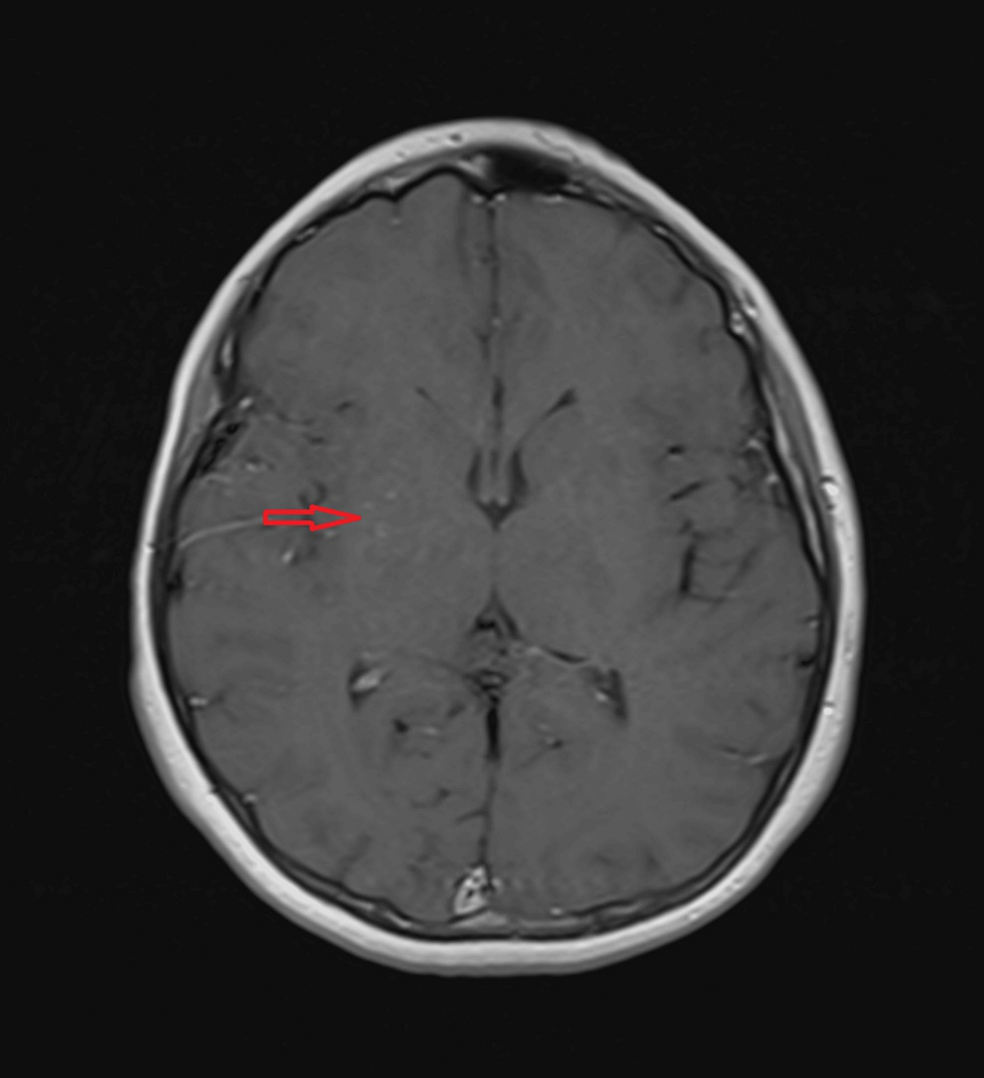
MRI, contrast-enhanced T1 weighted axial image showing faint collateral vessels (brush sign) in the right basal ganglia (red arrow)
